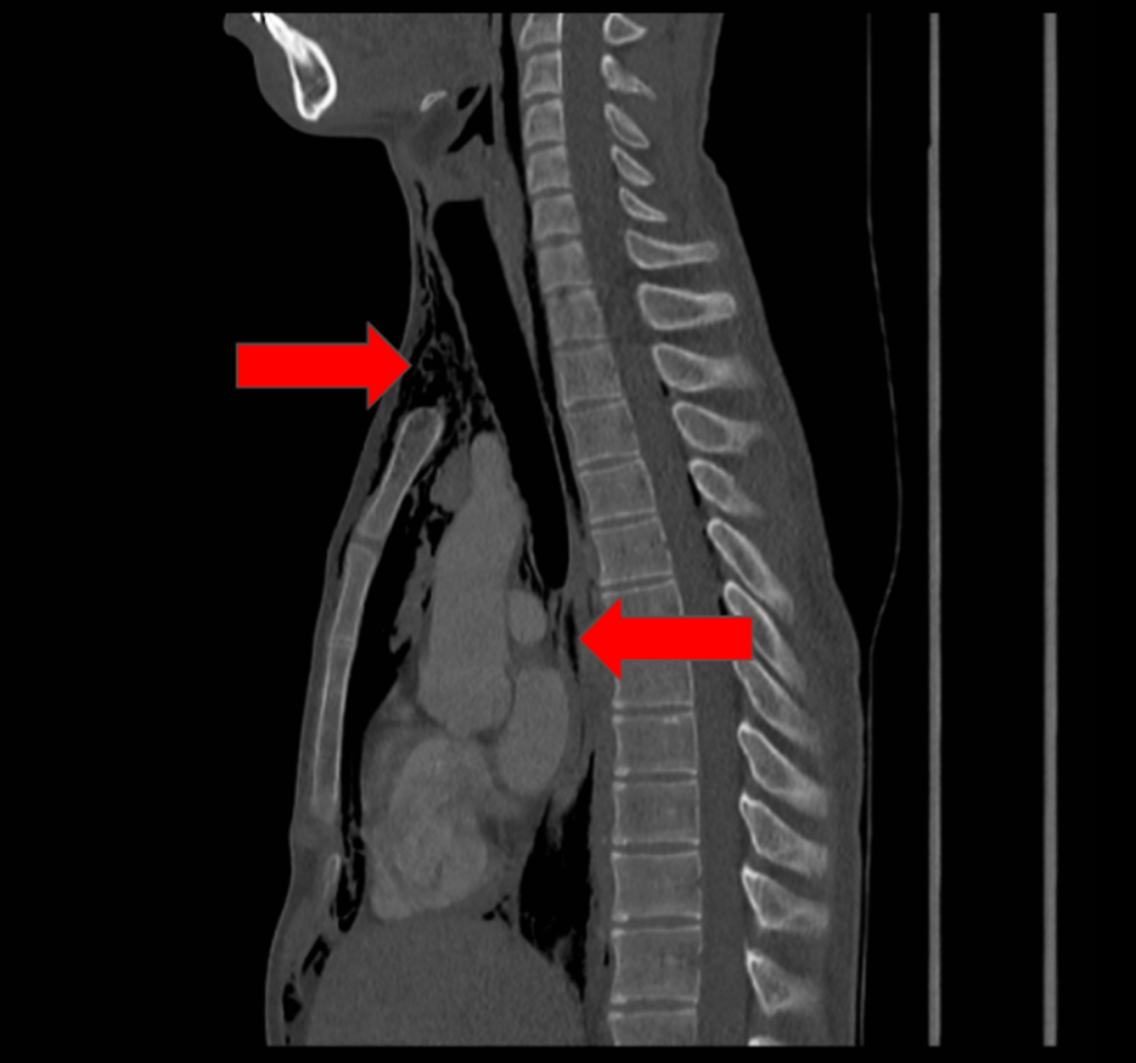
Sagittal computed tomography (CT) showing air in the mediastinum and soft tissues of the neck (red arrows)
